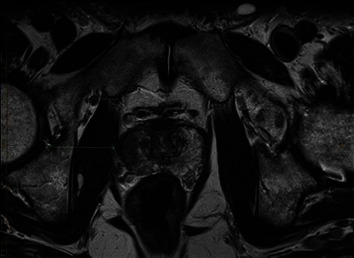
MRI image of prostate in patients with prostate cancer.
